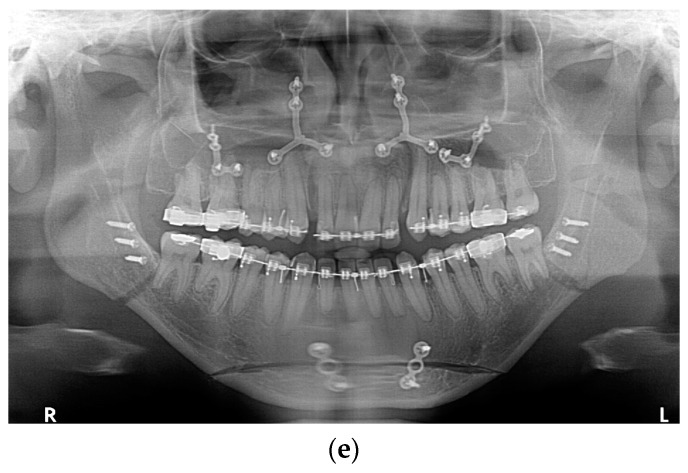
Panoramic X-ray with fixation hardware: (a) after BSSO; (b) after BSSO and genioplasty; (c) after Le Fort I osteotomy; (d) after bimax; (e) after bimax with maxillary segmentation and genioplasty.
